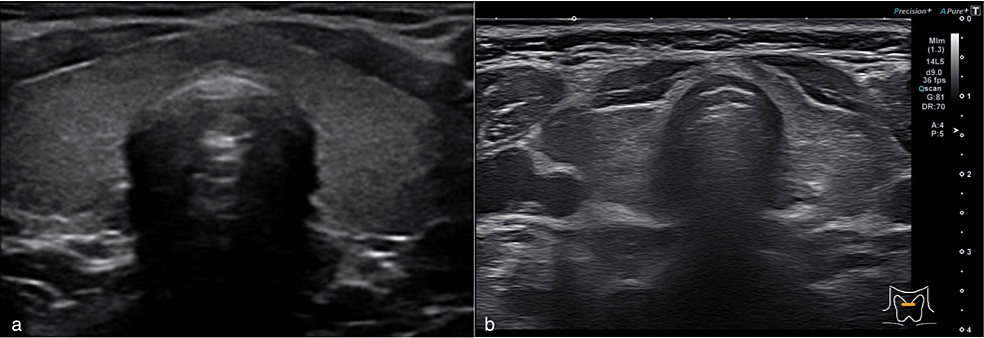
a) Ultrasound of normal thyroid gland with homogeneity. b) Ultrasound of our patient's thyroid gland showing evidence of slight heterogeneity. a): Source Ref. [6]
